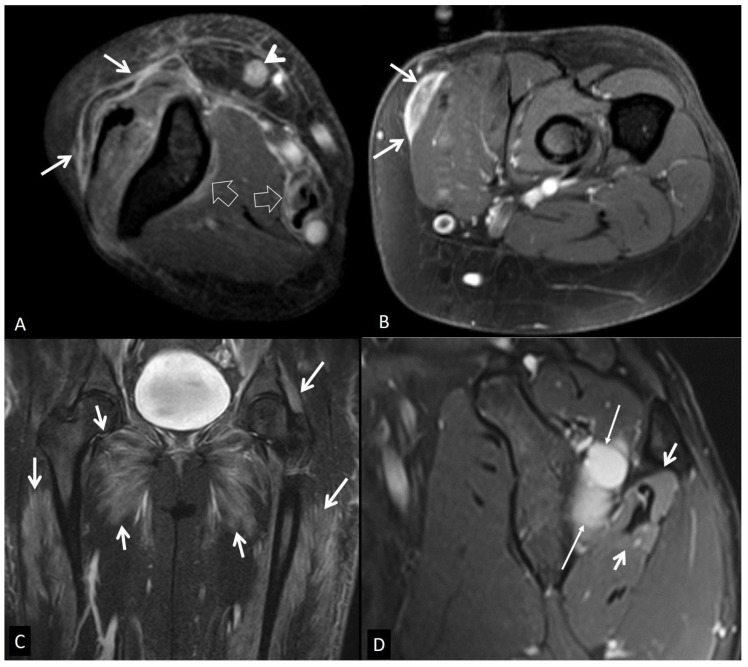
(A) Eosinophilic fasciitis in a 68-year-old female. Axial fat-suppressed contrast-enhanced T1-w MR images show enhancement of the superficial fasciae (arrows), deep fasciae (open arrows), and a small lymph node (arrowhead). (B) Nodular fasciitis in a 60-year-old female patient. Axial fat-suppressed contrast-enhanced T1-w MR image showing enhancement of the fascia (arrows). (C) Coronal STIR MR image in a 20-year-old female patient with dermatomyositis, showing diffuse muscle edema (arrows). (D) Muscle denervation in a 25-year-old male patient. Oblique sagittal PD-w MR image showing the ganglion cyst (thin arrows) and the edematous infraspinatus muscle (arrows). The rest of the rotator cuff muscles show normal signals.
